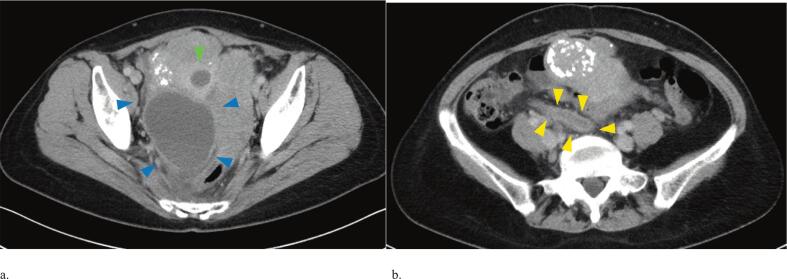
a. Computed tomography at admission showed an 8 cm right TOA (blue arrowheads), pyometra (green arrowhead). b. Computed tomography showed an enlarged appendix (yellow arrowhead). (For interpretation of the references to colour in this figure legend, the reader is referred to the web version of this article.)
